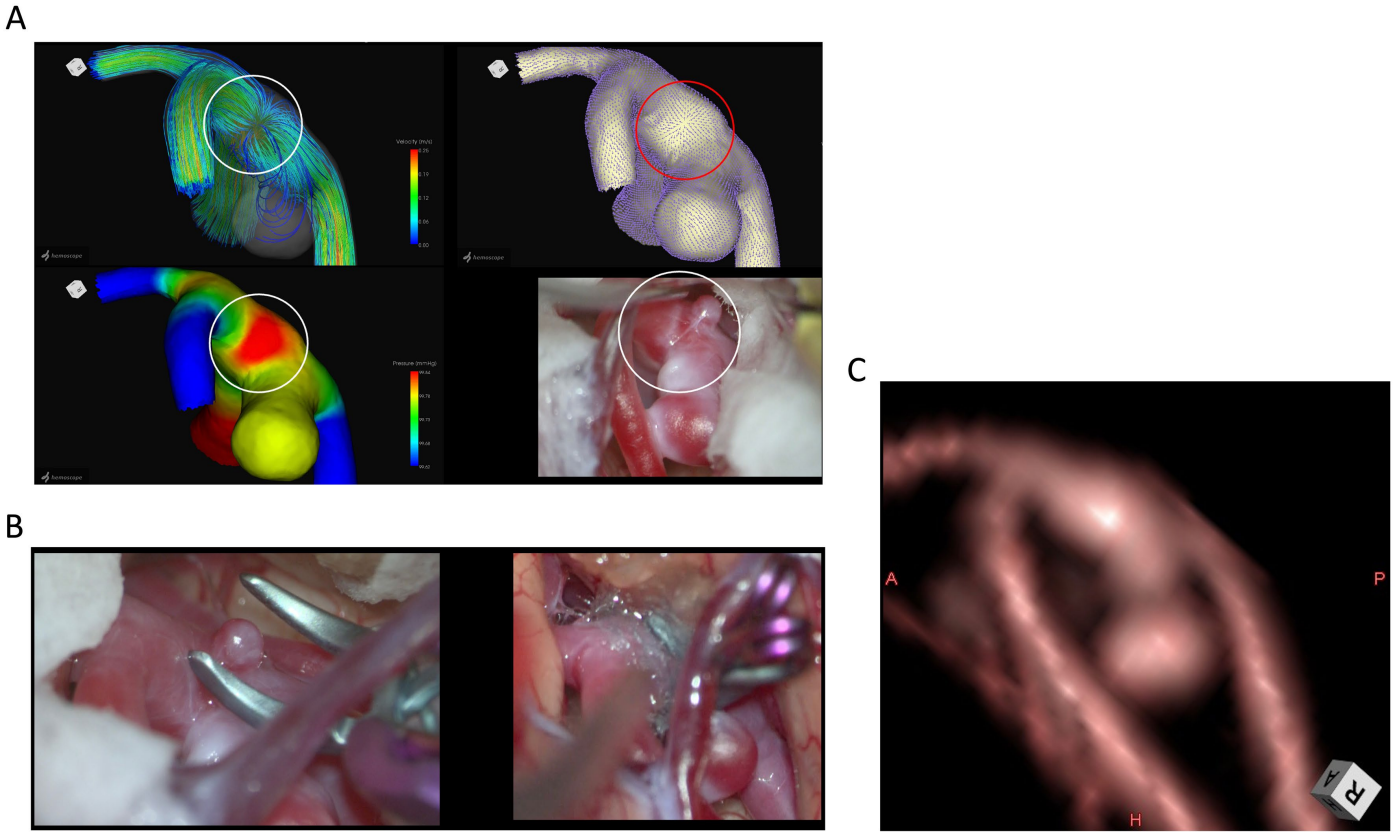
Case 2: A 70-year-old woman with a right MCA aneurysm. The CFD images and intraoperative view depict the radial dispersion of wall shear stress vectors and elevated wall pressure adjacent to the aneurysm within the encircled area (A). The intraoperative view of additional clipping for a microaneurysm adjacent to the aneurysm (B). Preoperative 3D-CTA image of the middle cerebral aneurysm at the angle of intraoperative findings (C).
